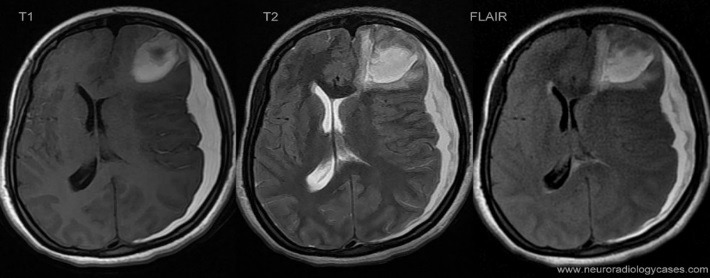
FLAIR, T1, T2 MRI images of an acute subdural haematoma of a left brain hemisphere which determines an important mass effect on the structures of the median line as well as the concomitant presence of an area of left frontal lobe laceration; complex brain injury
